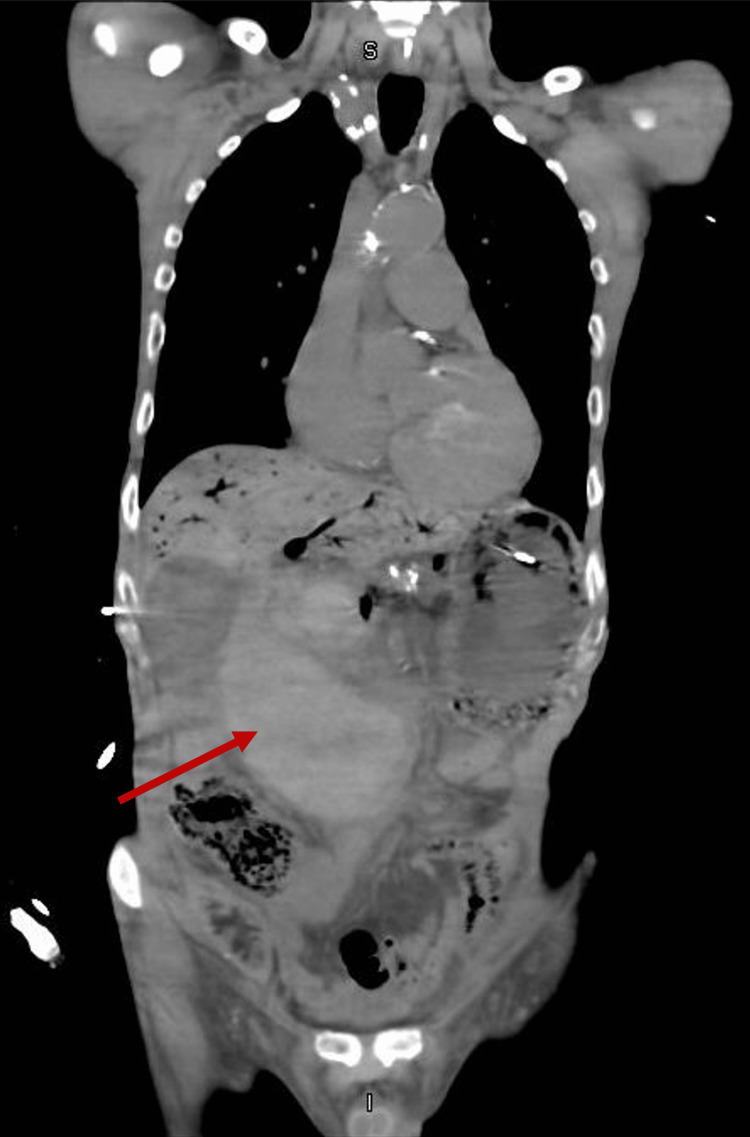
CT Abdomen/Pelvis (coronal view) The red arrow is showing the haematoma
